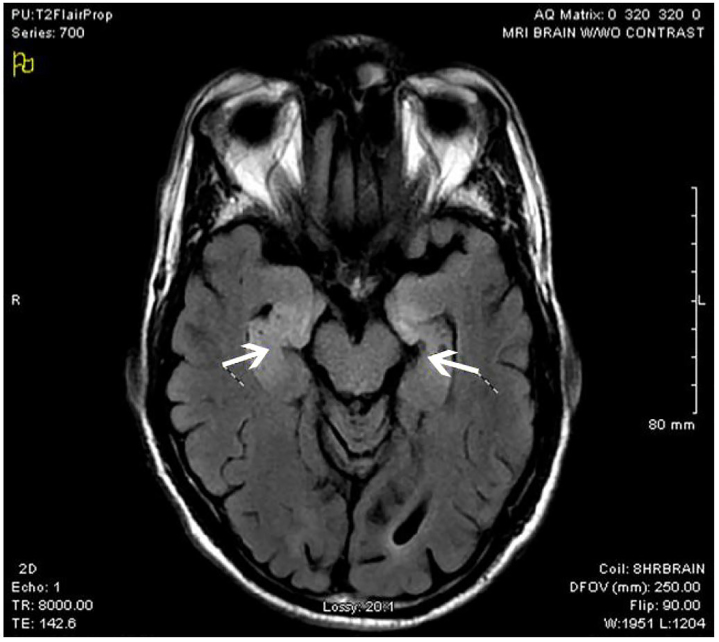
Axial T2 FLAIR sequence magnetic resonance imaging showing bilateral enhancement of the hippocampus (arrows). *FLAIR,* fluid attenuated inversion recovery
